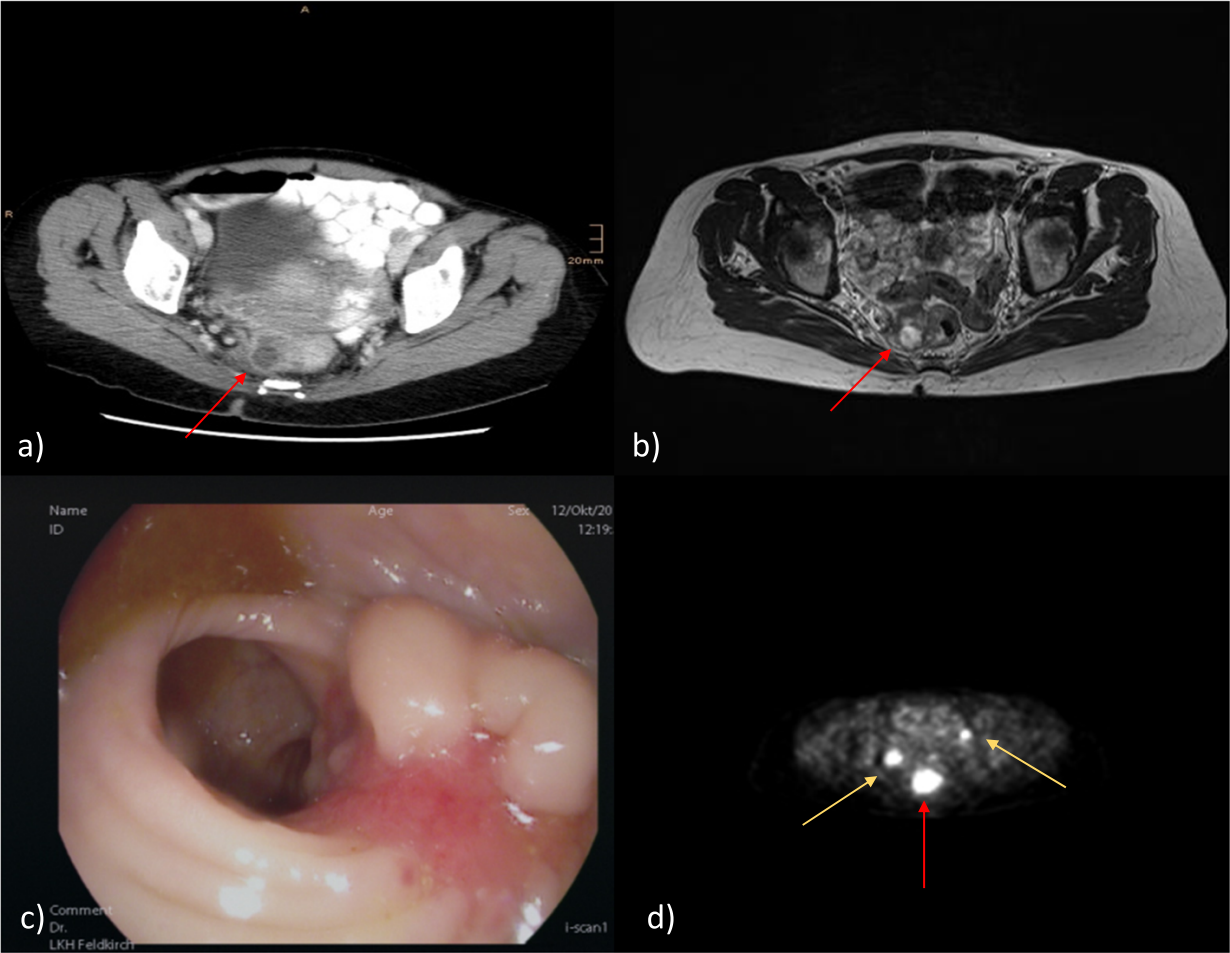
Preoperative imaging. a Computed tomography scan. b Magnetic resonance imaging axial. c Endoscopy. d Positron emission tomography scan. In computed tomography scan and magnetic resonance imaging only, primary tumor was clearly detected (*red arrow*). A cystic ovary was described. To exclude foreign metastasis a positron emission tomography scan was performed with an enhancement in both ovaries (*yellow arrows*)
